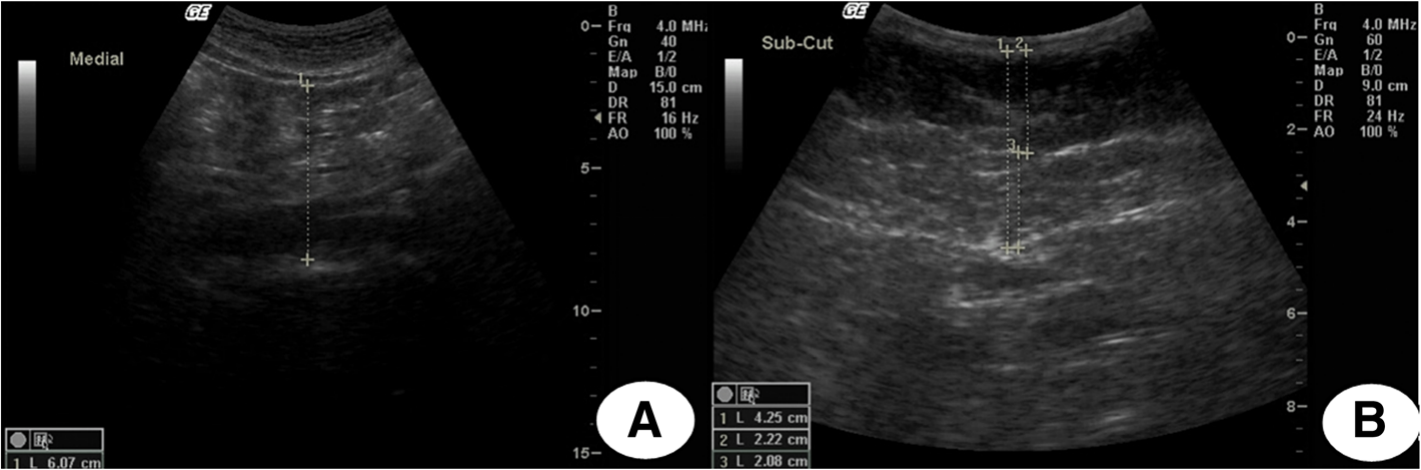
Ultrasound image examples to abdominal adipose tissue measurement. a visceral adipose tissue thickness. b subcutaneous adipose tissue thickness and components (deep and superficial)
